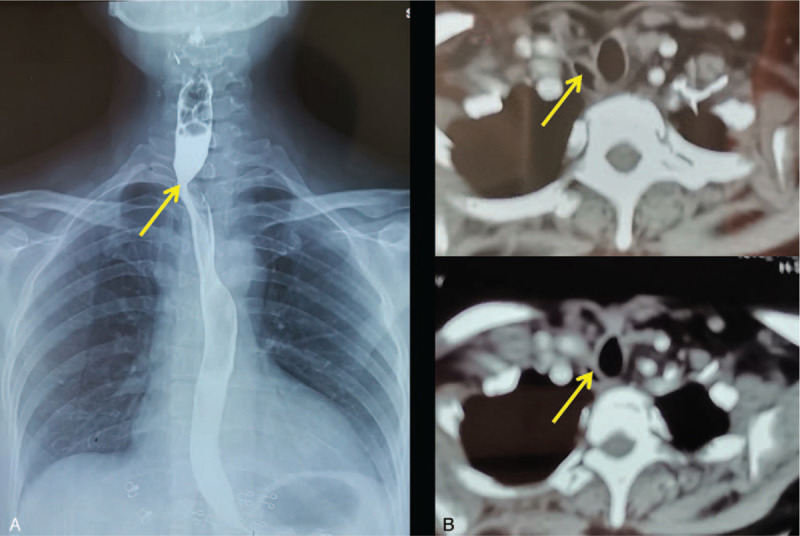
Postoperative esophagography and CT scan. A: Esophagography shows the function of reconstructed esophagus at the third month postoperatively; B: CT shows the reconstructed esophagus at the second year postoperatively. Note: reconstructed esophagus (yellow arrow).
